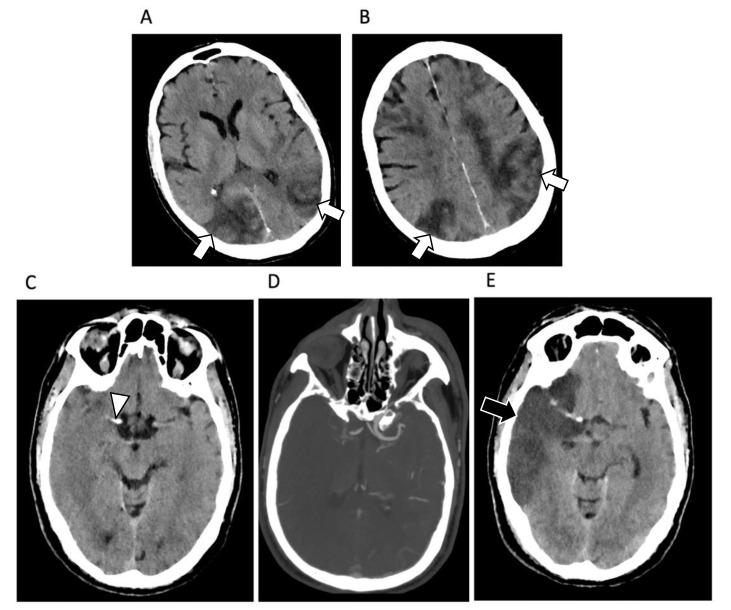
Brain findings in COVID-19 patients. (A,B) Axial brain CT images of a 56-year-old man affected by SARS-CoV-2-related pneumonia showing multiple hypoattenuating areas located in the cortical junction bilaterally, with a predominant extension in the parietal and occipital lobes, due to ischemia (white arrows). (C) Axial brain CT images of a 71-year-old woman affected by SARS-CoV-2-related pneumonia showing hyperdense artery sign in the right middle cerebral artery (white arrowhead), due to acute thrombosis. This finding was confirmed by brain CT angiography (D) showing a complete thrombosis of the middle cerebral artery. After 15 days brain CT images (E) show a large hypoattenuating area in the fronto-parietal lobe (black arrow) due to the brain ischemia.
